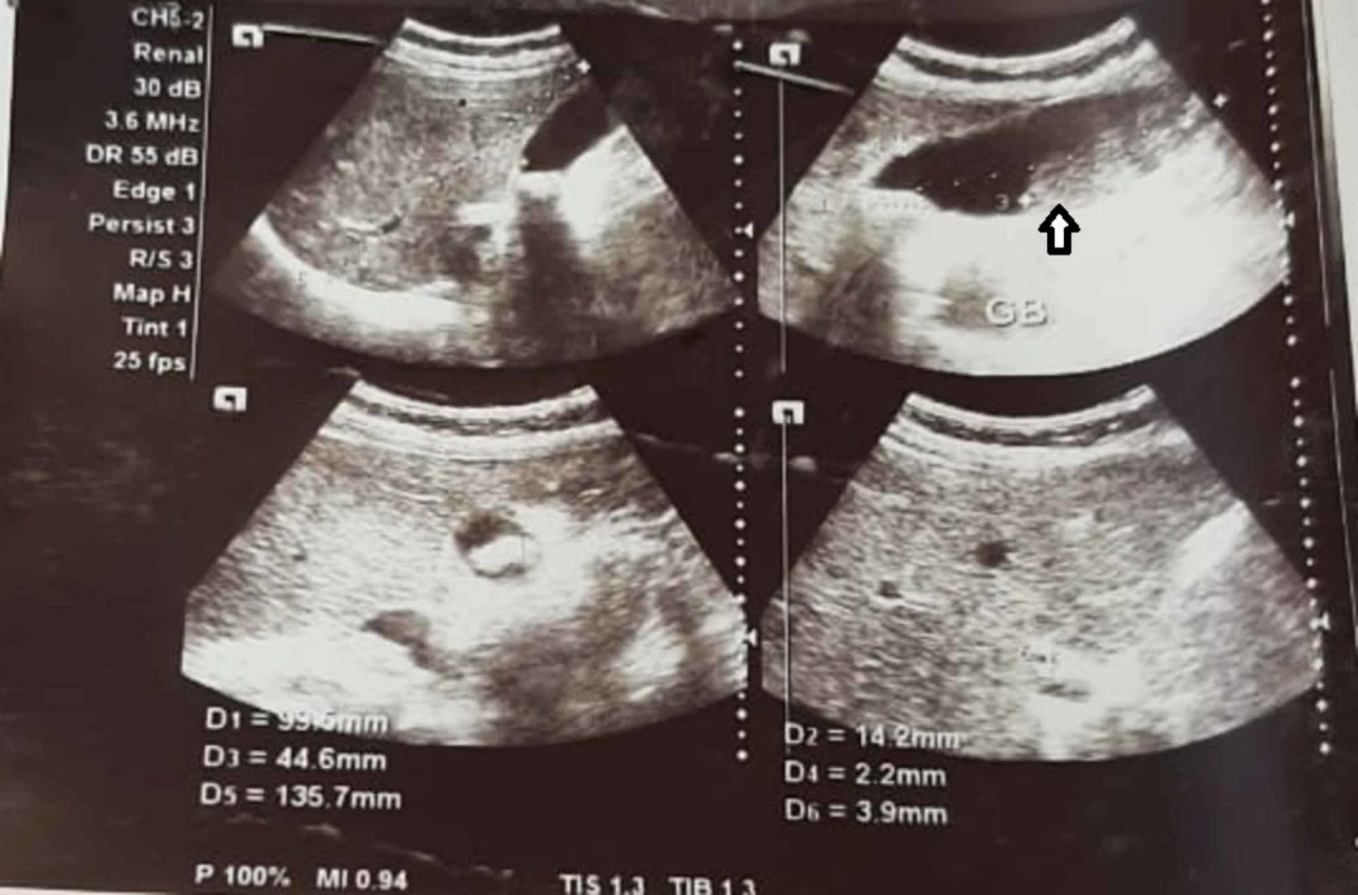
Ultrasound abdomen and pelvis with the arrowhead showing the distended gall bladder with multiple calculi with sludge
